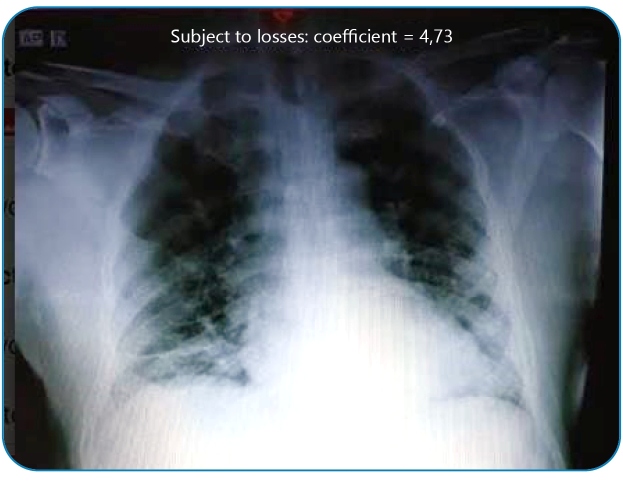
Chest radiography on the 10th day of symptoms.
